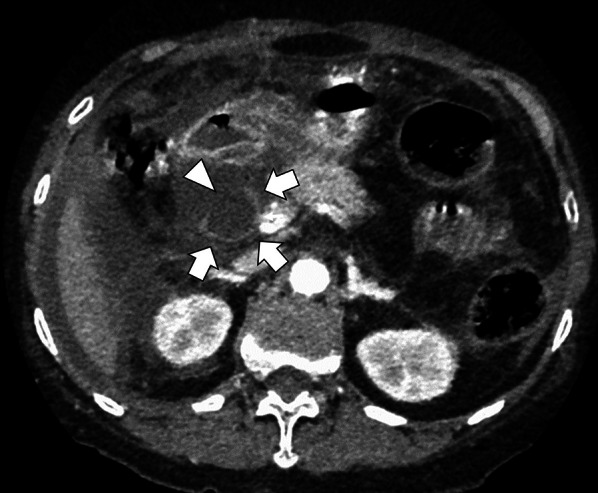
Preoperative computed tomography (axial view). Enhanced computed tomography showed a dilated common bile duct (arrow) with a partial defect in continuity (arrowhead) and peritoneal fluid around the liver
